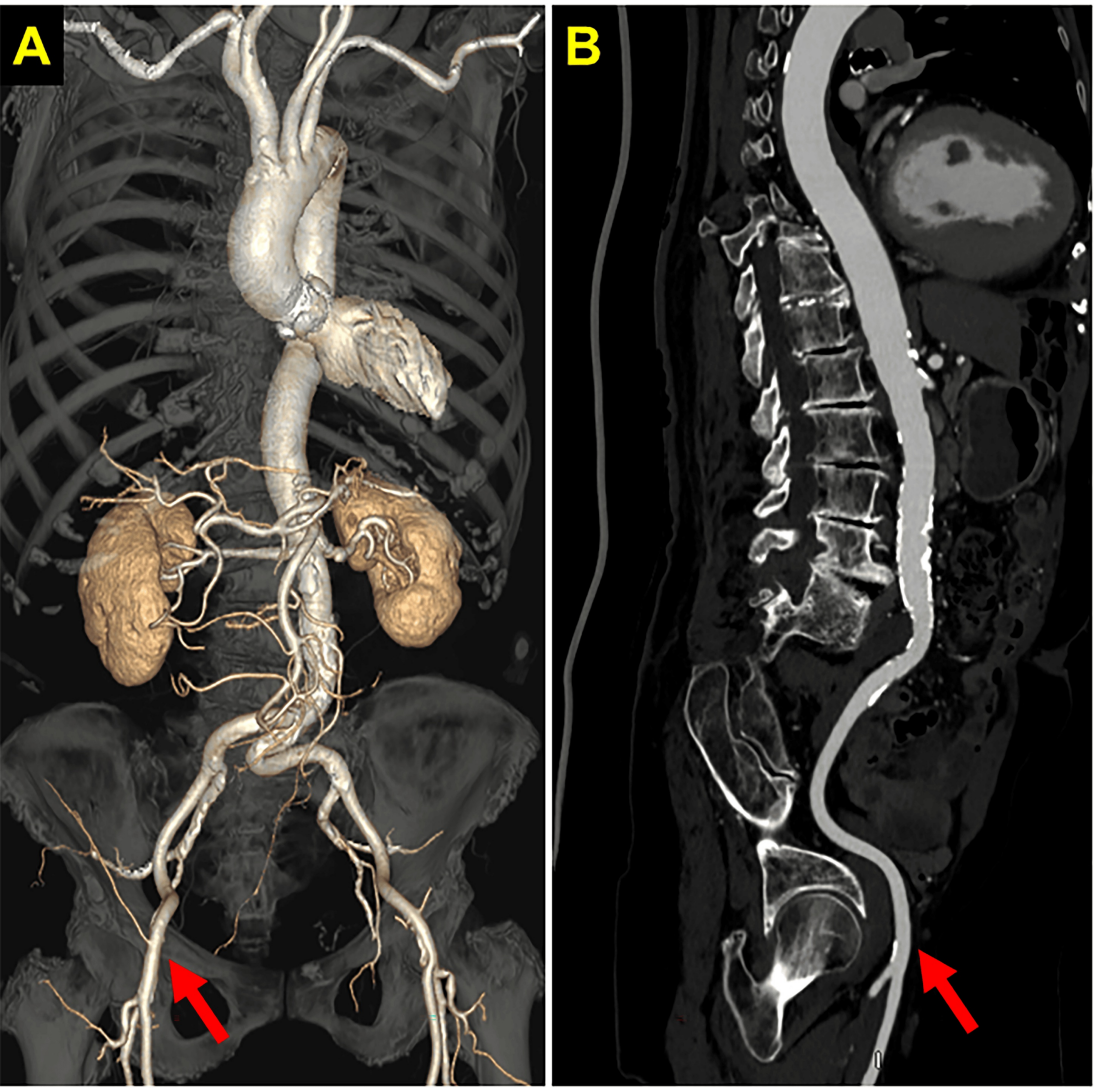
Findings of pre-procedural enhanced computed tomography Red arrows indicate the access site.
